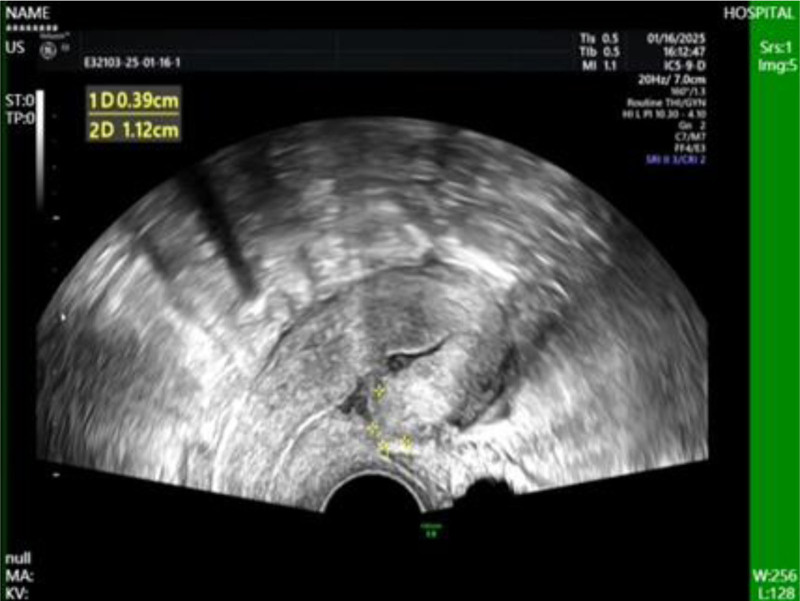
Postoperative transvaginal ultrasound showing a localized hypoechoic area.
